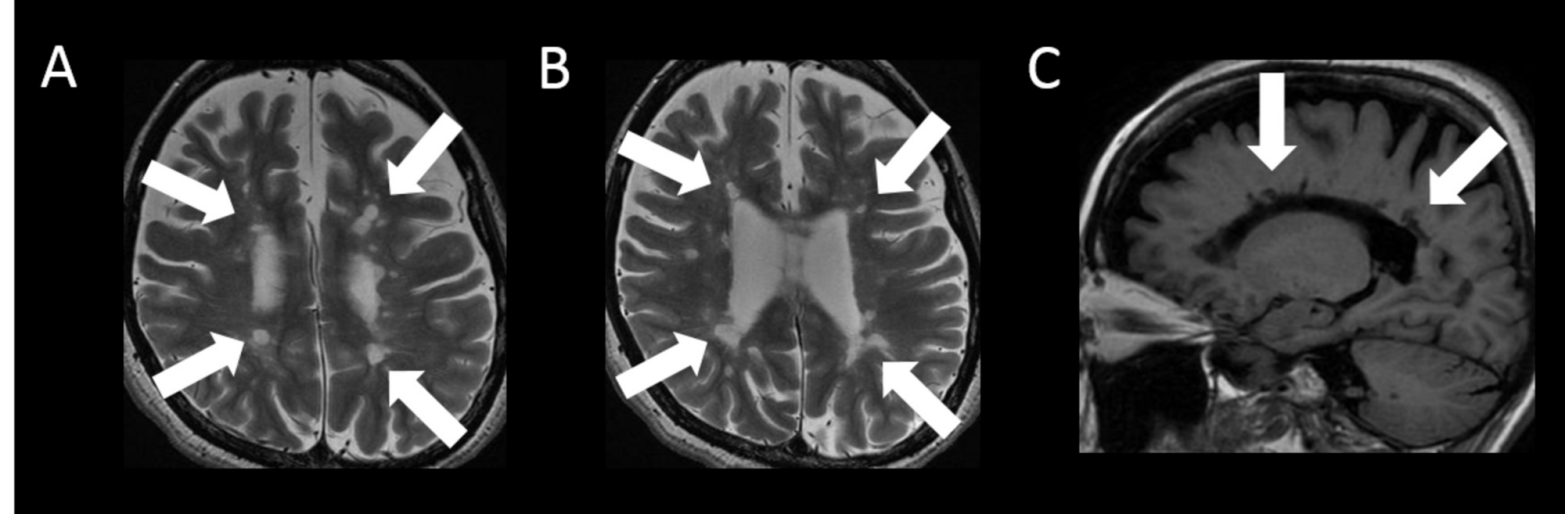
Brain MRI findings - lesions in the periventricular and subcortical white matter A, B: brain T2-weighted imaging shows high-intensity lesions in the periventricular and subcortical white matter (white arrows); C: T1-weighted imaging shows that many of these lesions are hypointense (white arrows) MRI: magnetic resonance imaging
